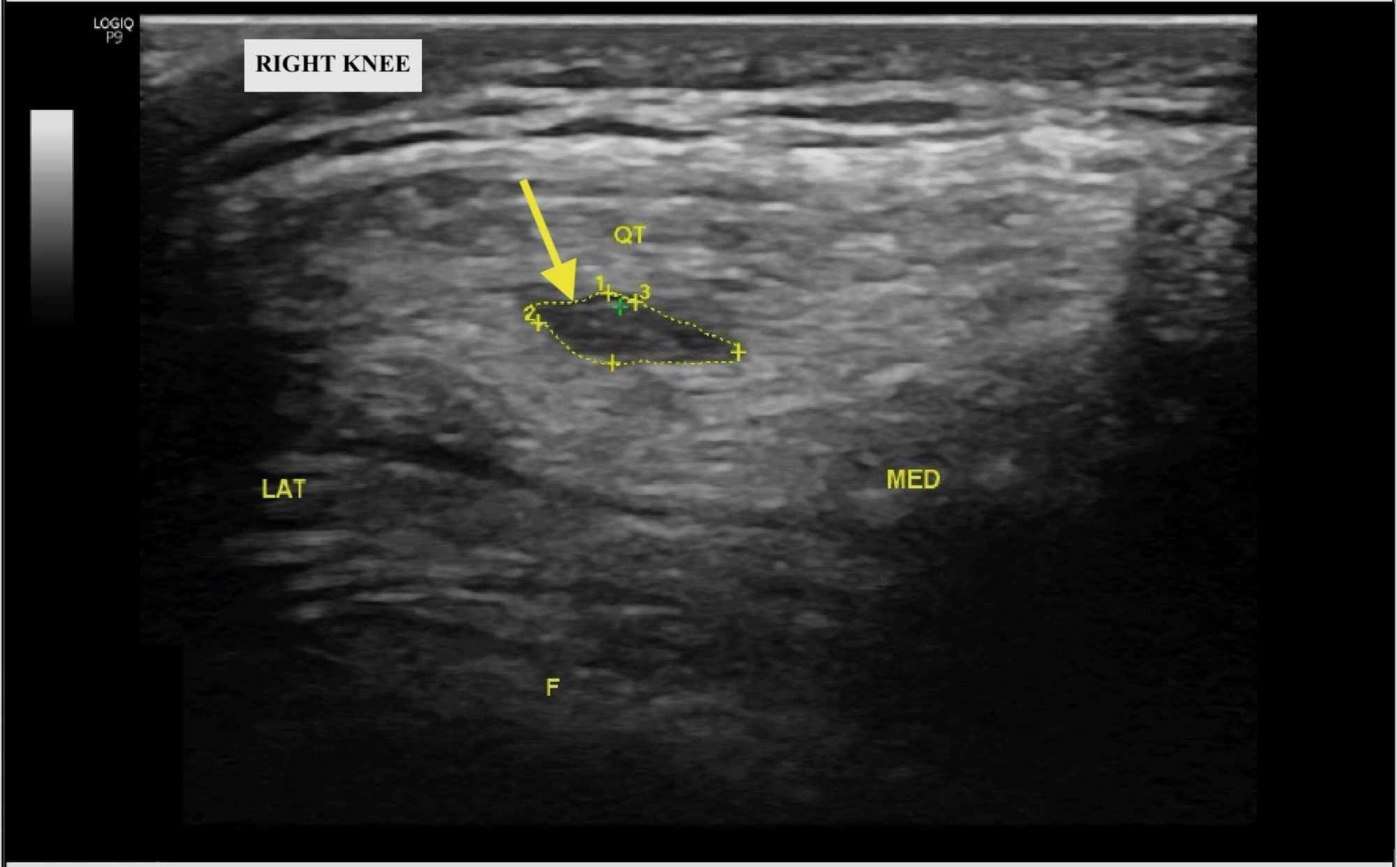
Short-axis ultrasound image of the right knee at three-month follow-up demonstrating hypoechogenicity within the right quadriceps tendon (yellow arrow) LAT: lateral anatomic orientation; F: femur; MED: medial anatomic orientation; QT: quadriceps tendon
